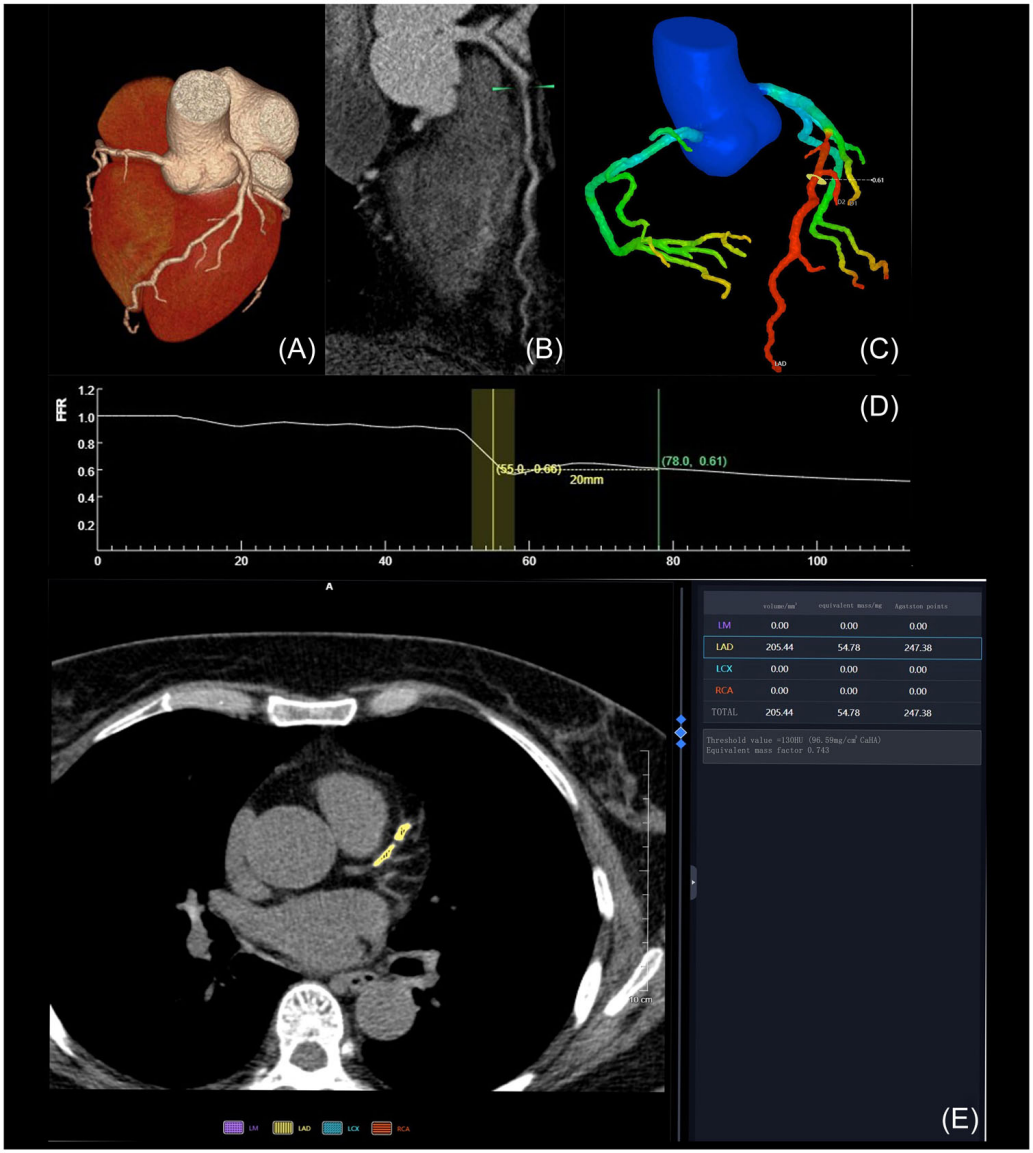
(A) Cardiac volume rendering image (VR); (B) computed tomography angiography curved planar reformation image; (C) VR coronary tree color map; (D) CT fractional flow reserve value curve; (E) coronary artery calcification score calculation interface.
